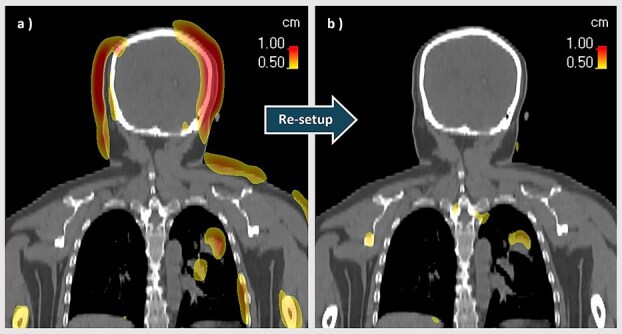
Results of deformable image registration from the fraction with the largest observed setup error. (a) Uncorrected kVCT for planning CT. (b) Re-setup of kVCT for planning CT. The color map represents the amount of deformation.
